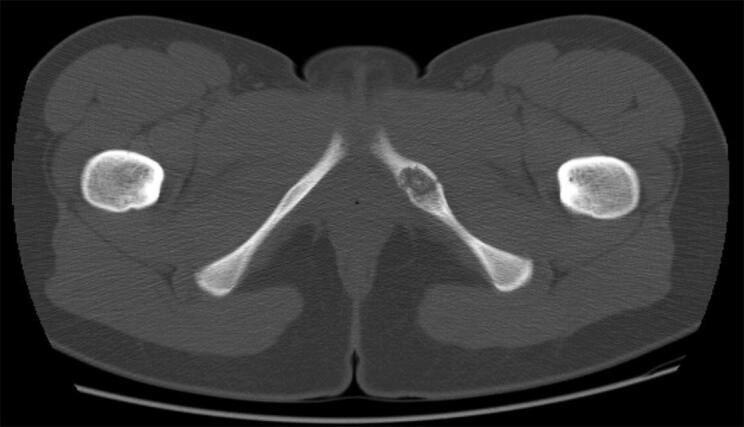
CT scan showing enlargement of the left IPS in a 16-year-old female patient with VND.
